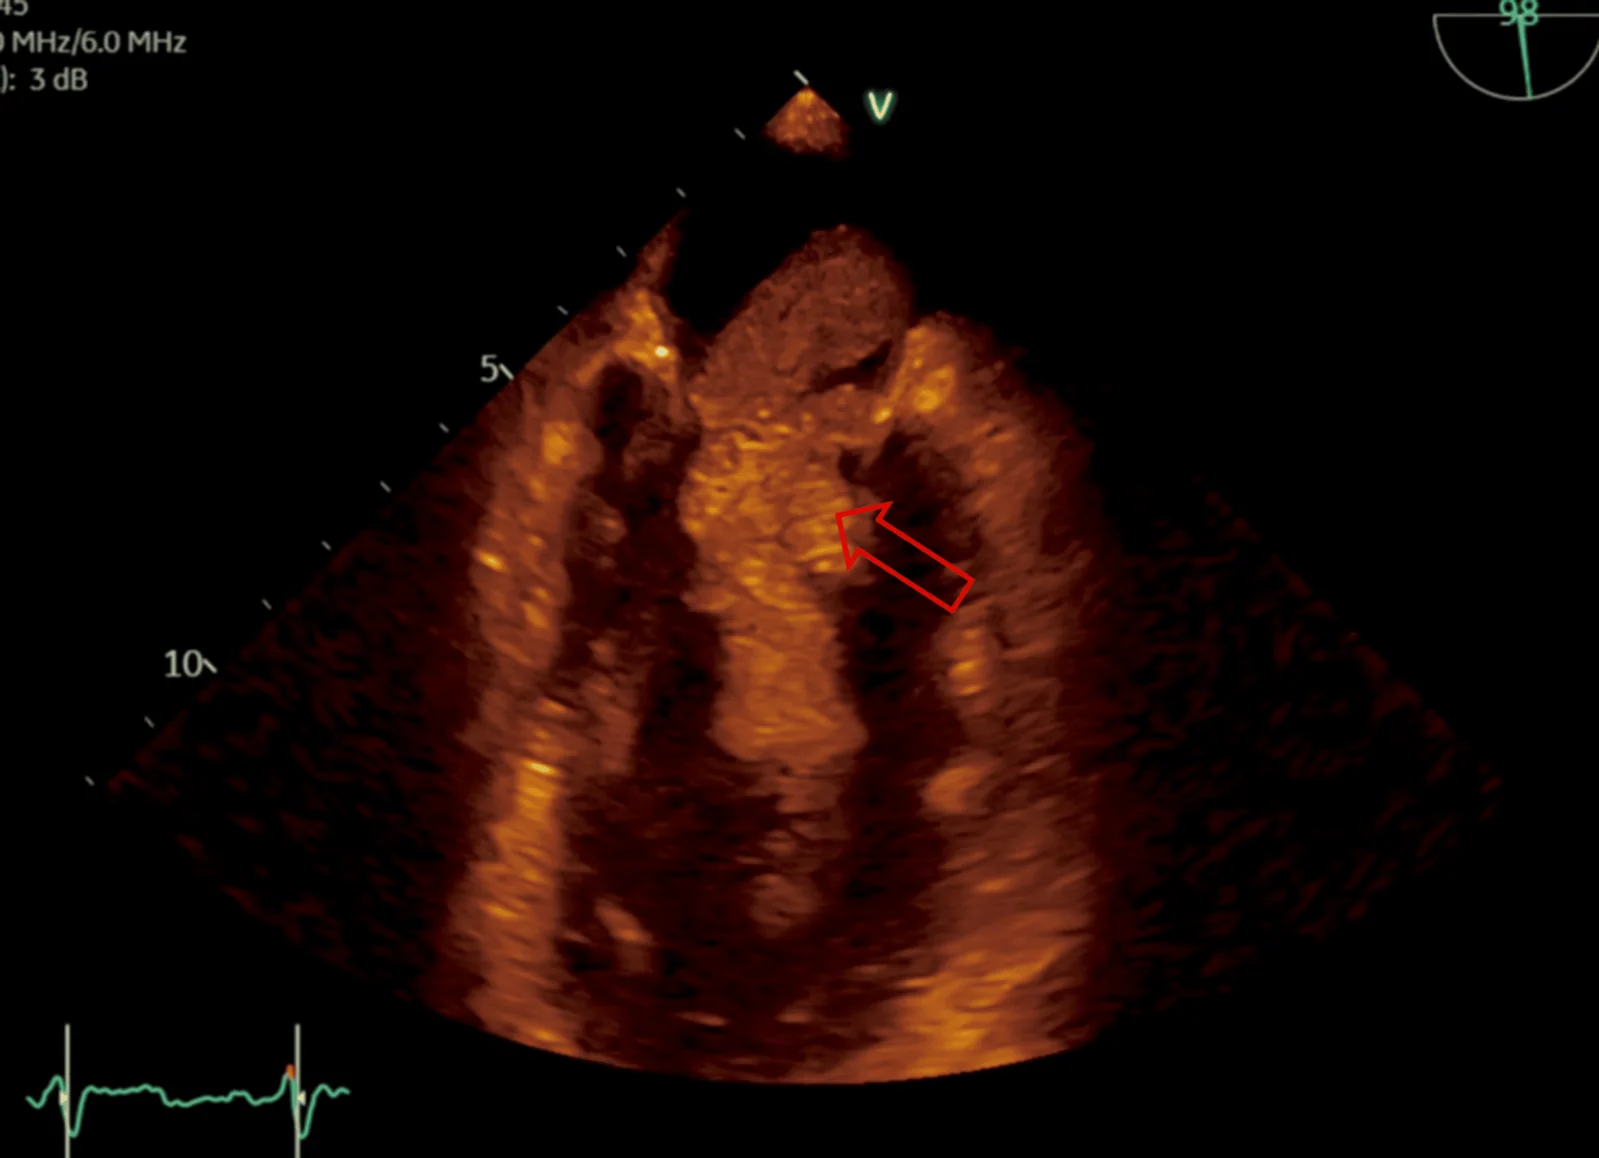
Transesophageal echocardiography (mid-esophageal two-chamber view), demonstrating a giant multilobulated left atrial mass prolapsing into the left ventricle.
